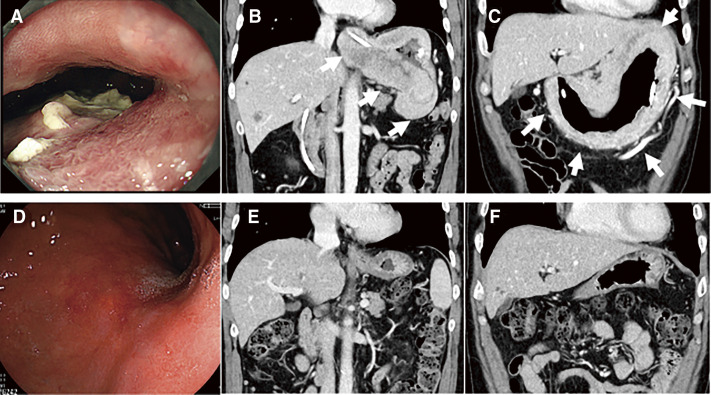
Case presentation. (A) Gastroscopy demonstrated large type 4 gastric cancer, and biopsy confirmed poorly differentiated adenocarcinoma. (B) and (C) were abdominal CT images (coronary view), and they showed that this huge tumor invaded not only the whole stomach but also the distal esophagus (arrows). This patient received S-1-based chemotherapy using an ED tube. The tumor shrank dramatically, and the ED tube was removed only after 2 weeks from the start of the treatment. After 1 year of the treatment, gastroscopy demonstrated the complete disappearance of the tumor (D), and no cancer cells were seen in the biopsy samples. Abdominal CT images after 1 year of the treatment also demonstrated the complete disappearance of the tumor (E, F). After 2 years of chemotherapy, this patient completed the chemotherapy and survived more than 80 months from the start of the chemotherapy without recurrence. ED, elementary diet
